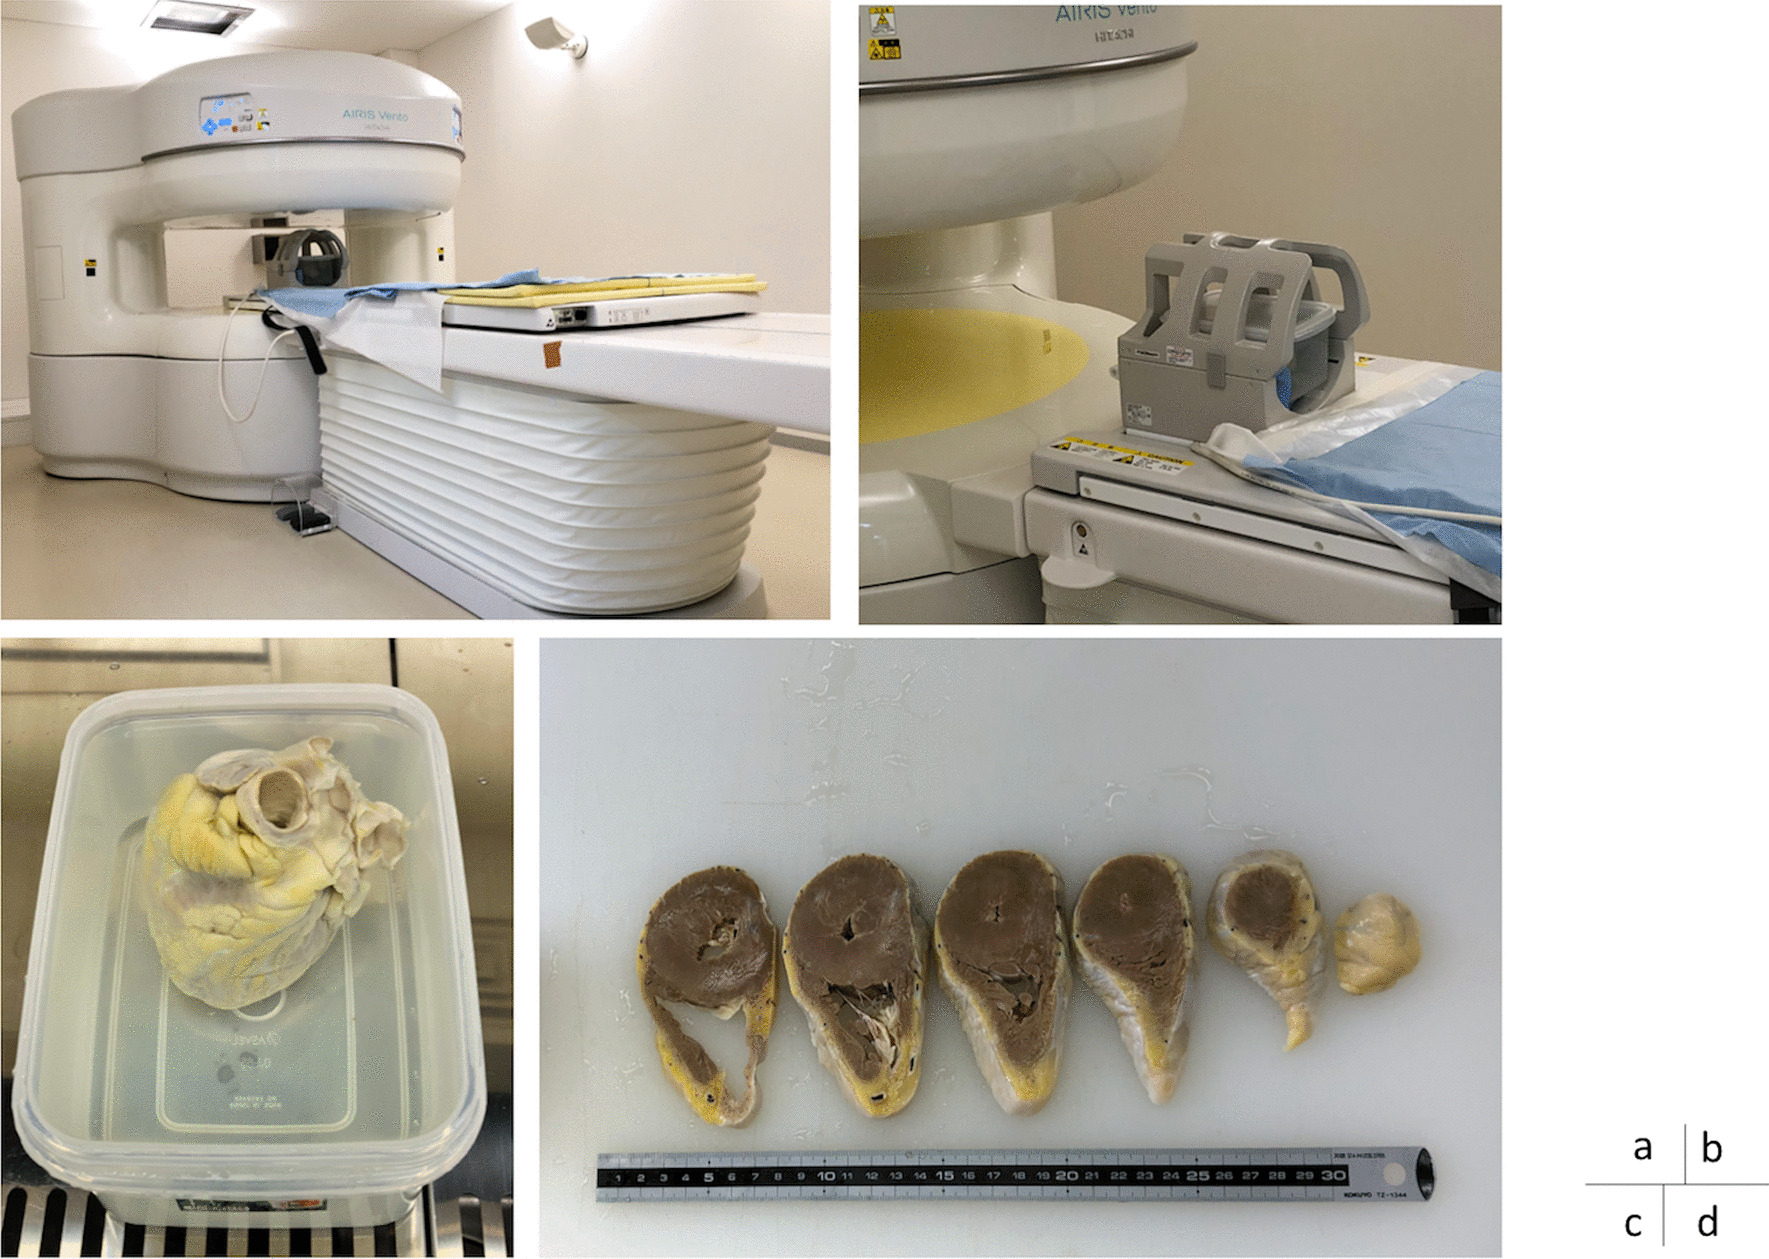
a External view of the MRI scanner. b QD coil. c Formalin-fixed heart. d Sliced heart
